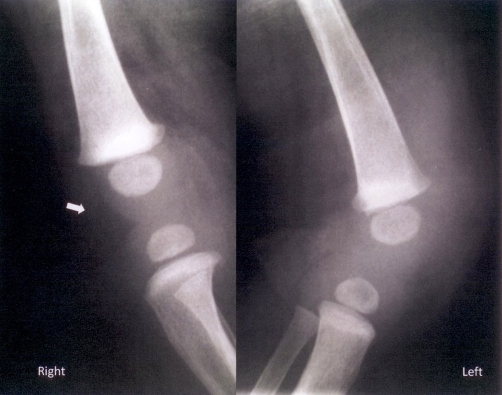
Plain radiograph in lateral projection of both knee joints. Indistinctness of soft tissue-fat iterface at the left knee joint is demonstrated suggesting presence of oedema. Note the normal right side with clear fat plane (arrow) imaging. There is also a radiolucent area at the left tibial epiphysis suggesting osteomyelitis.
